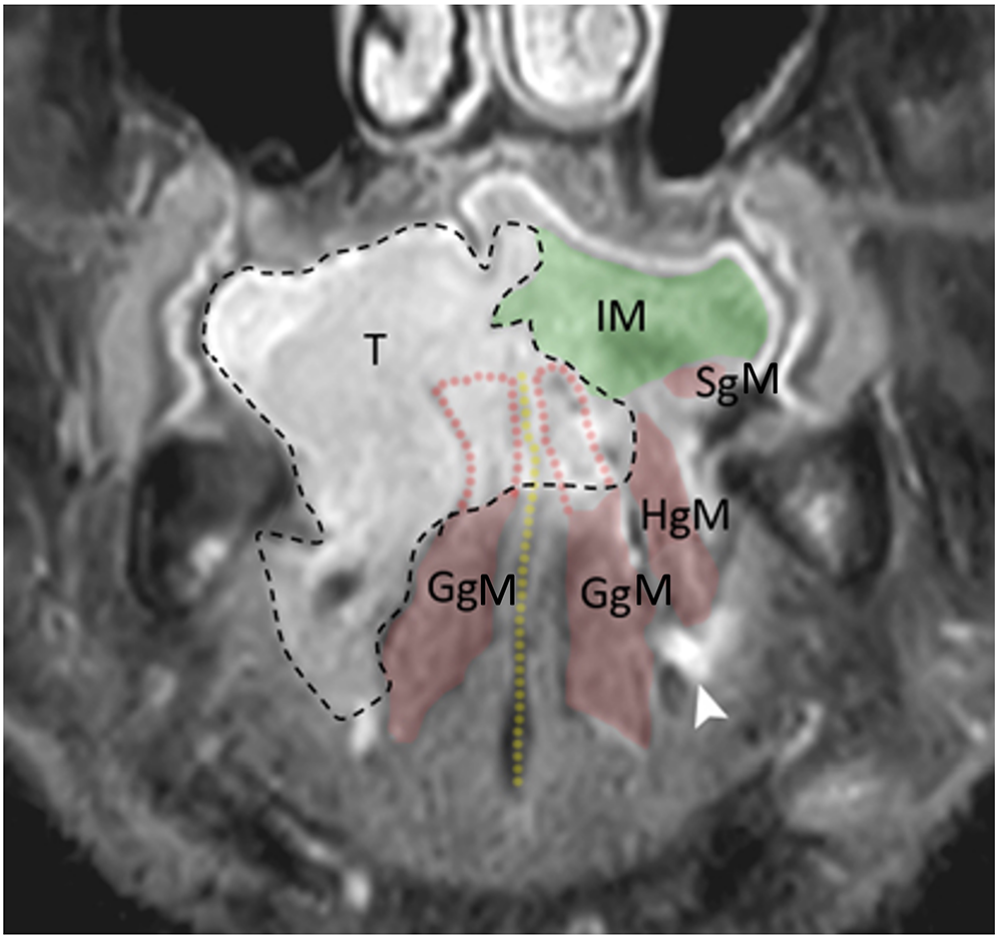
MRI sagittal view: tumor enveloping the genioglossus muscle on both sides. T, tumor; IM, intrinsic musculature; SgM, styloglossus muscle; GgM, genioglossus muscle; HgM, hyoglossus muscle. Yellow dotted line, lingual septum; black dotted line, tumor border.
